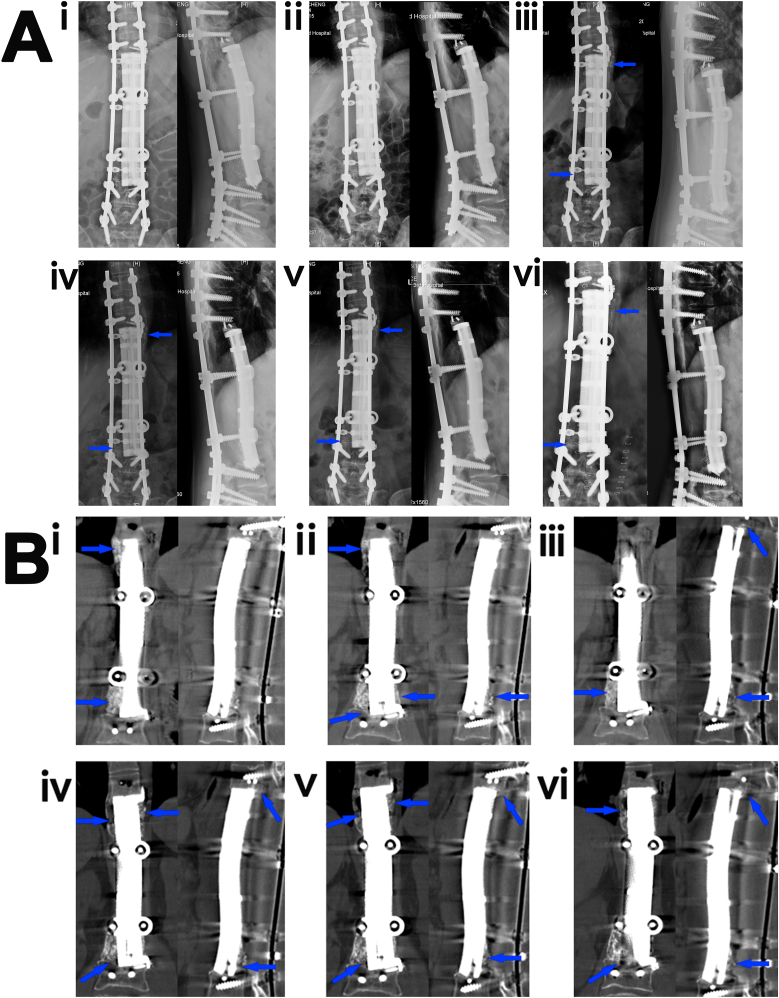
A spine defect reconstructed by a three-dimensional (3D)-printed porous Ti6Al4V implant (Case 1). (A) (i-vi) Radiographs of the reconstructed 19-cm spine defect at 1 (i), 3 (ii), 7 (iii), 12 (iv), 24 (v), and 32 (vi) months after implantation. Blue arrows indicate the newly formed bone in the implant-bone interface or on the outer surface of the implant. (B) Computed tomography images of the reconstructed 19-cm spine defect at 3 (i), 7 (ii), 12 (iii), 28 (ⅳ), 32 (v), and 36 (vi) months after implantation. Blue arrows indicate the newly formed bone in the implant-bone interface or on the outer surface of the implant.
